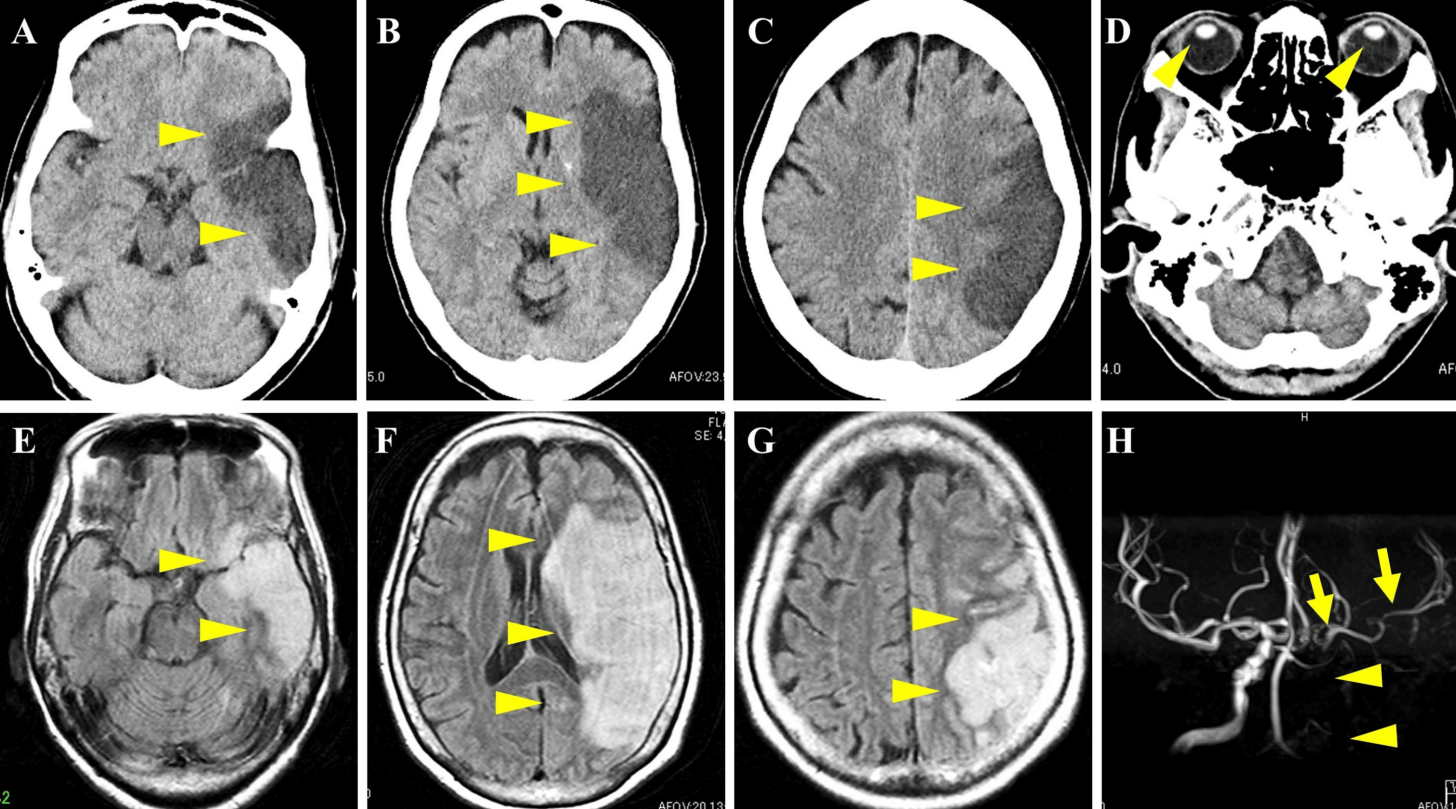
CT, MRI, and MRA of the brain CT (A-C) and MRI (E-G) showed extensive infarction of the left middle cerebral artery (arrowheads) without brain edema or herniation. The midline shift was minimal, and minor basal ganglia calcification was noted (B). MRA showed occlusion of the left internal carotid artery (D; arrowheads), but the left middle cerebral artery is faintly apparent from crossflow (H; arrows). Note that the frontal eye field (Brodmann’s area 8) and visual cortex (Brodmann’s area 39) were intact CT: computed tomography, MRI: magnetic resonance imaging, MRA: magnetic resonance angiography
